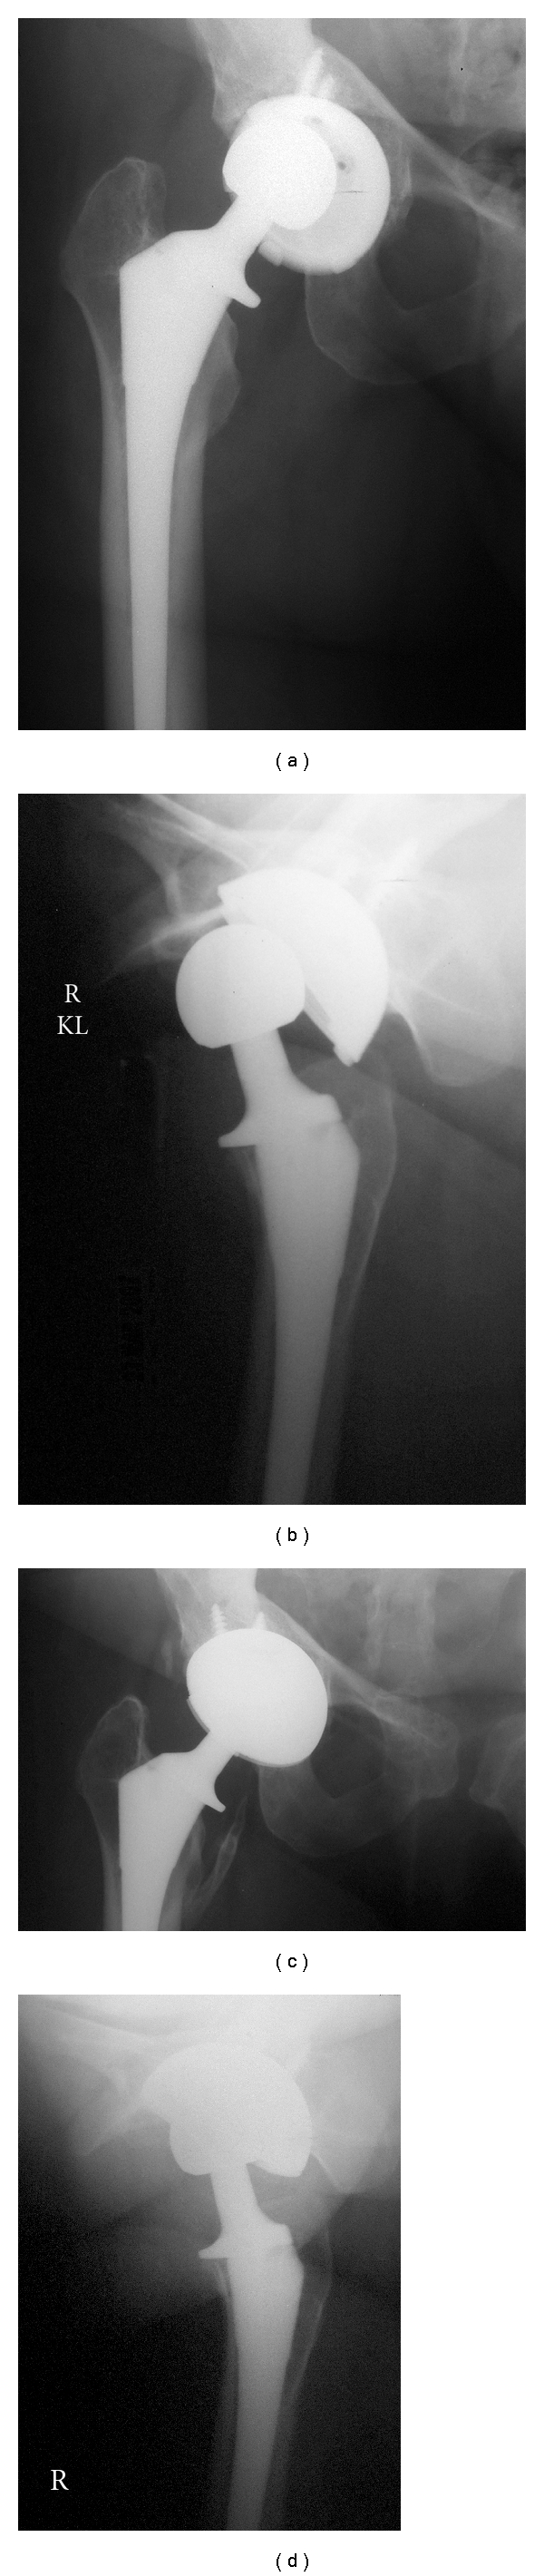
(a) Patient 1: anteroposterior radiographs demonstrating eccentric placement of the femoral head within the acetabular polyethylene and excessive acetabular component abduction. (b) Patient 1: direct lateral radiograph demonstrating anterior femoral head subluxation and excessive acetabular component anteversion. (c) Patient 1: anteroposterior radiographs demonstrating concentric femoral head positioning and corrected acetabular component abduction. (d) Patient 1: direct lateral radiograph demonstrating concentric femoral head positioning and corrected acetabular component abduction.
